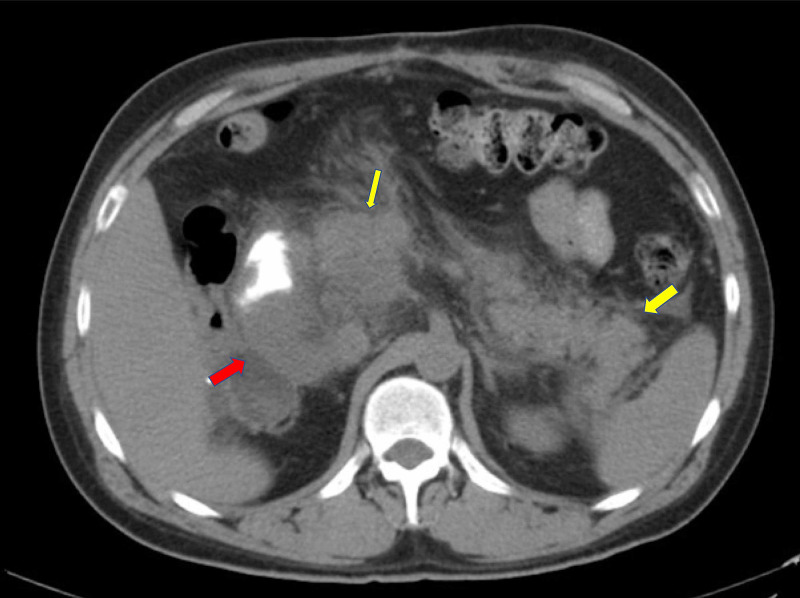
CT scan of the abdomen and pelvis transverse plane. Right retroperitoneal mass (red arrow). Acute pancreatitis with stranding involving the head of the pancreas (yellow thin arrow) and tail (yellow thick arrow). Pancreatic ducts are not visualized.
